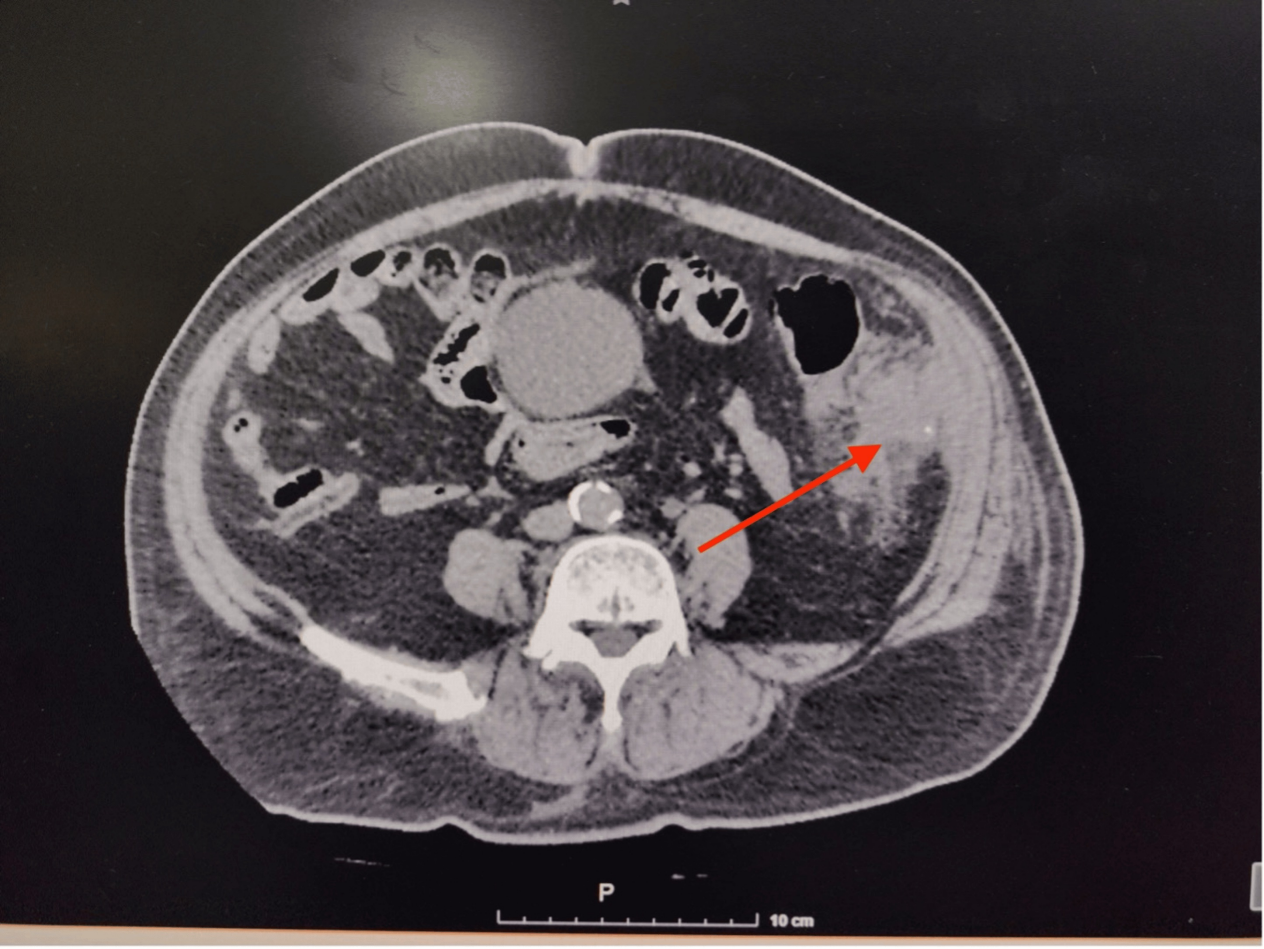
CT scan revealed a mass adjacent to the descending colon with peripheral enhancement dependent on the colonic wall, extending into the muscular layer, suggestive of an abscess, as well as a small radio-opaque object in the middle of the phlegmon, supportive of a foreign body
